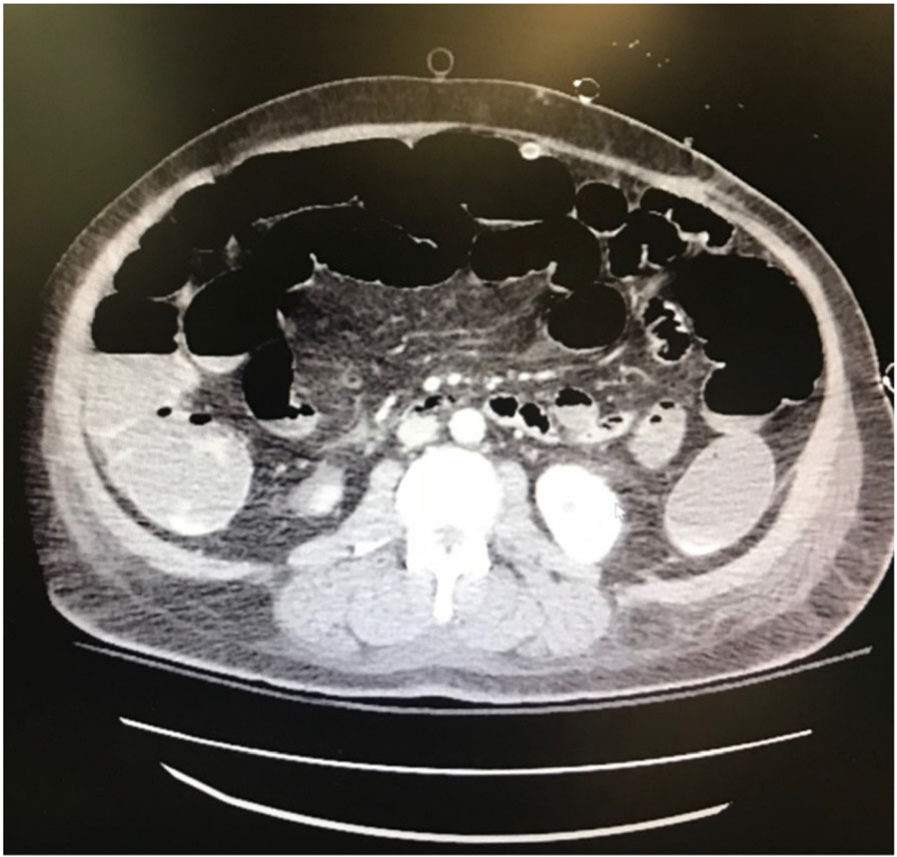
CT Abdomen revealing air-fluid levels, with dilation of multiple loops of bowels, as well as decompressed loops concerning for small bowel obstruction
